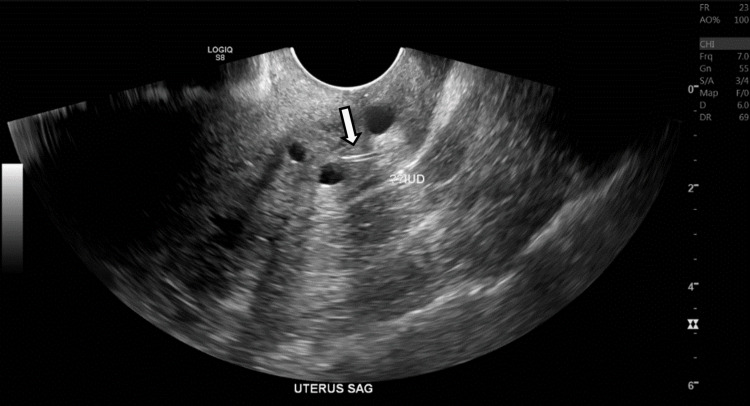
Transvaginal Ultrasound Showing Suspected IUD Fragment
